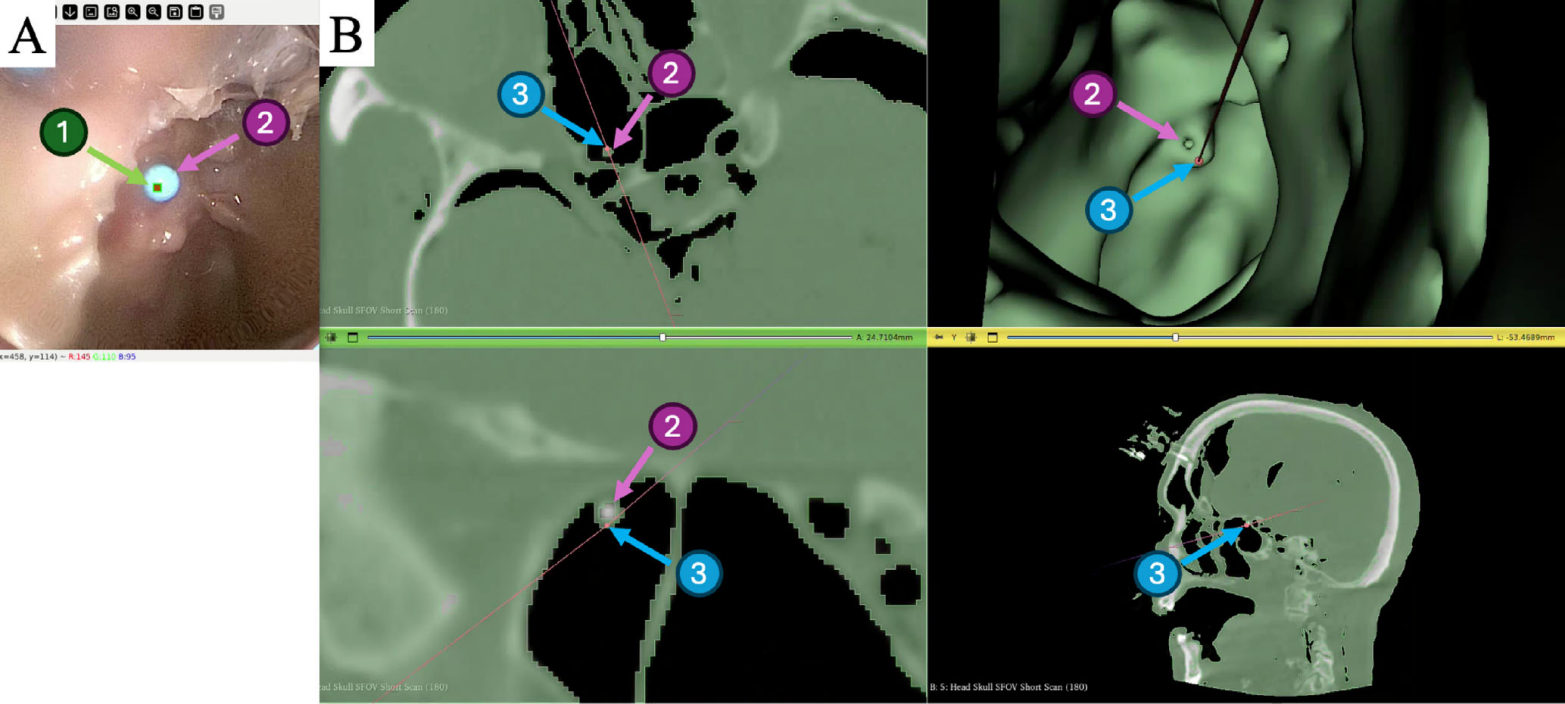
(A) Image endoscope reference view, (B) axial, coronal and sagittal views of the explored anatomy, and a view of the CT segmentation. (1) shows a visual reference in the image, centered on a fiducial marker (2). (2) is also highlighted in the CT scan. (3) represents the projection of the visual reference (1) onto the CT scan.
